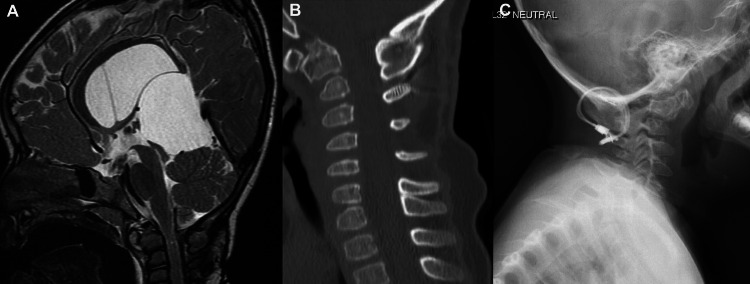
Last follow-up. (A) T2-weighted brain MRI shows significant improvement of the craniocervical junction narrowing along with focal atrophy and myelomalacia at the C1-C2 level. (B) The craniocervical junction CT demonstrates bony fusion with posterior assimilation at the occiput through C3 with intact cerclage wires. (C) Neck X-ray reveals a persistent increase in C1 and C2 vertebral body sclerosis with residual mild anterior translation. The atlantodental interval is significantly narrowed as well. MRI, magnetic resonance imaging
